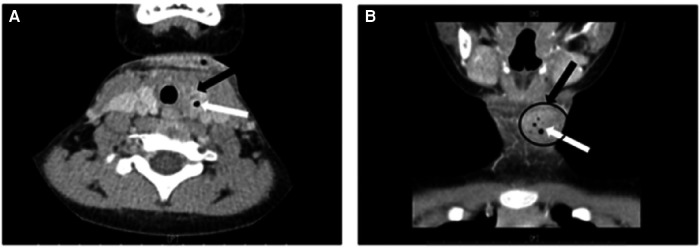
CT scan of neck. (A) Axial view; (B) coronal view. Black arrow and black circle: irregular low-density mass in the left lobe of the thyroid, consistent with a deep cervical abscess near the left thyroid; white arrow: point-like gas density shadow.
